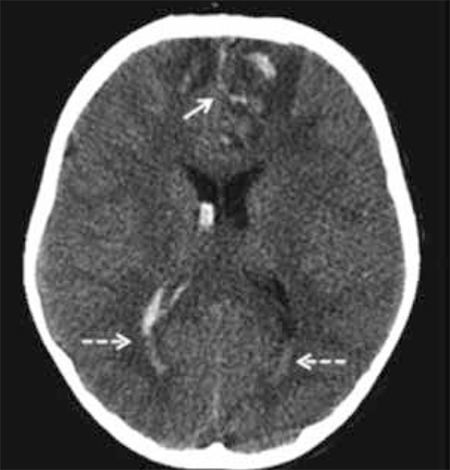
Axial nonenhanced computed tomography image shows hemorrhagic contusion areas in the frontal lobes bilaterally as foci of hyperdensity involving the grey matter and subcortical white matter and hyperdensities filling the subarachnoid space due to subarachnoid hemorrhage in the anterior interhemispheric fissure and sulci in the frontal lobes (arrow). In addition, intraventricular hemorrhage is seen (dashed arrow)
